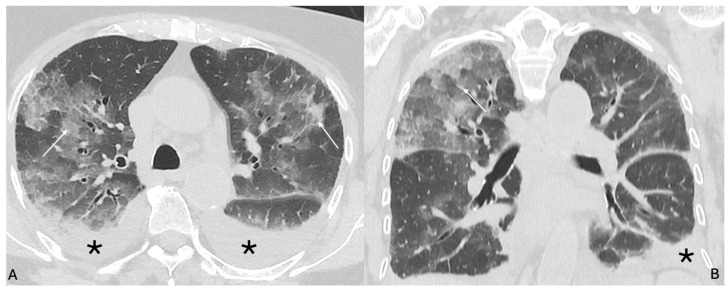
Axial non-contrast chest CT (A) and coronal reformatted image (B) demonstrate bilateral, predominantly upper-lobe ground-glass opacities with a perihilar distribution. At the level of the apical segments—particularly in the right upper lobe (white arrows)—a superimposed crazy paving pattern is evident, characterized by ground-glass attenuation associated with interlobular septal thickening and fine intralobular reticulation. Bilateral pleural effusions are present (asterisks), more pronounced on the left, with associated dependent posterior basal atelectatic consolidation. In the appropriate clinical context, these findings are suggestive of lipoid pneumonia; however, differential diagnoses including pulmonary oedema, infectious pneumonia, and other interstitial processes should also be considered.
